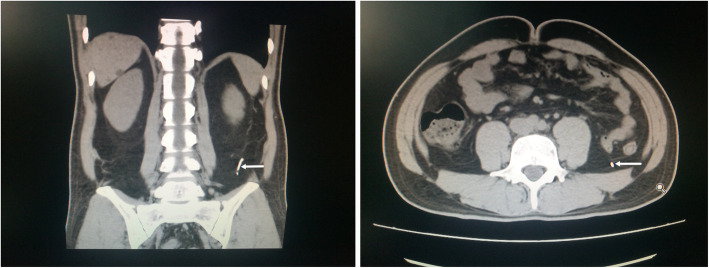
Preoperative Abdominal CT. The broken acupuncture needle was showed by arrow
